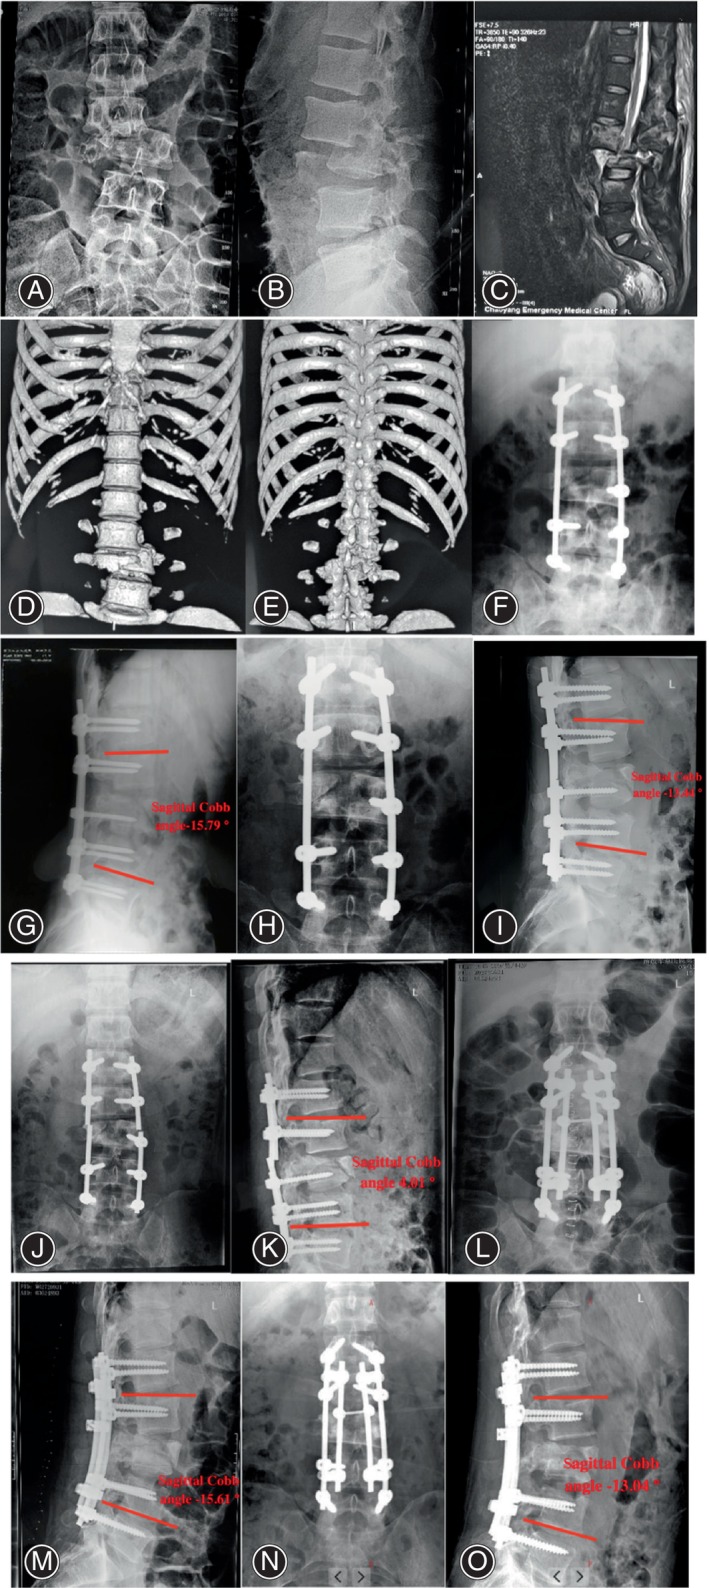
A 43‐year‐old man with lumbar 3 fracture and dislocation (ASIA A). (A–E) Preoperative X‐ray, CT, and MRI. (F, G) anterior and lateral position immediately after the first operation, sagittal Cobb angle −15.79°; (H, I) the left side was fractured 11 months after the first operation; the sagittal Cobb angle was –13.44°. (J, K) the right side was fractured 14 months after the first operation. Sagittal Cobb angle was 4.01°. (L, M) the sagittal Cobb angle was −15.61° immediately after revision; (N, O) during the 2‐year follow‐up, the sagittal Cobb angle was −13.04°, no fracture rod and no loss of kyphosis.
